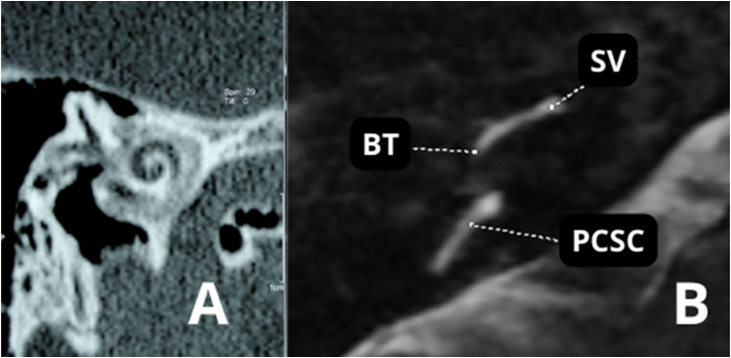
(A) Advanced otosclerosis with double ring/halo sign. (B) MRI ‒ T2-weighted sequence of the same patient showing stenosis of the scala tympani in the basal turn. BT, Basal Turn; SV, Scala Vestibuli; PCSC, Posterior Semicircular Canal.
